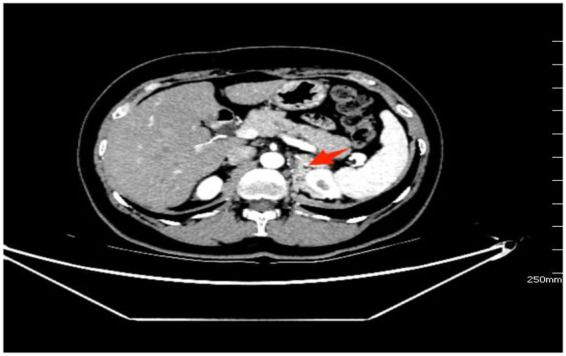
Enhanced abdominal CT scan in January 2021 showing the left adrenal gland.
